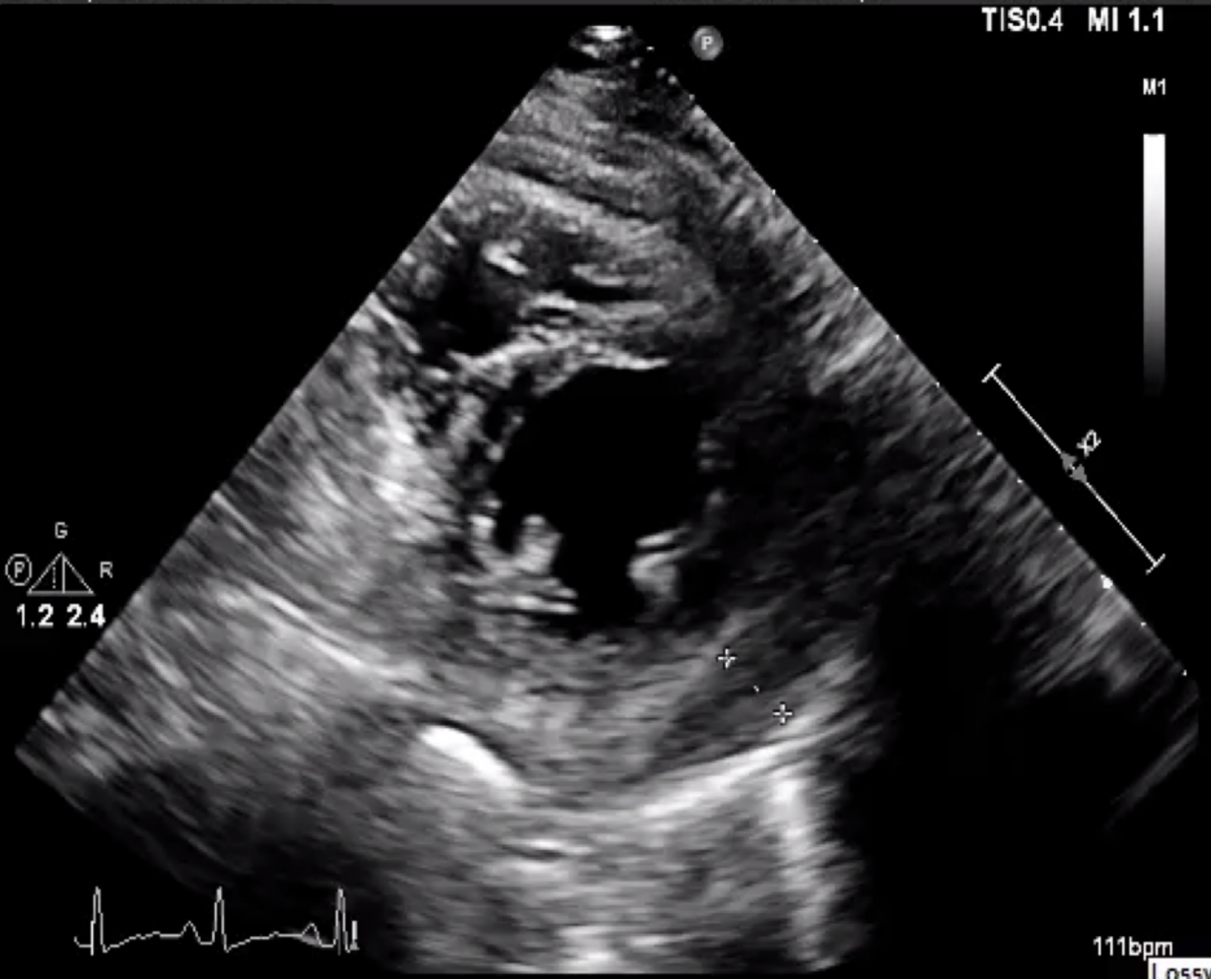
Follow-up transthoracic echocardiogram (parasternal short axis view) after three months upon discontinuation of clozapine showing resolution of the pericardial effusion
